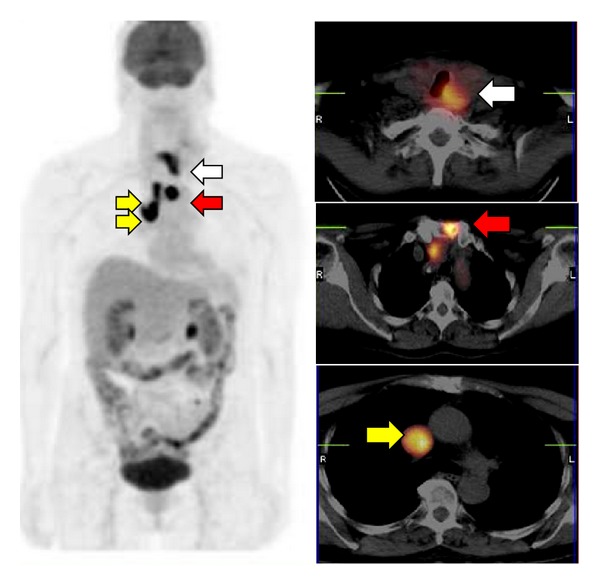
FDG-PET/CT in a 58-year-old female with ATC showing the presence of increased uptake in the thyroid tumour (white arrow) and sternal (red arrows) and mediastinal lymph nodal metastases (yellow arrows).
